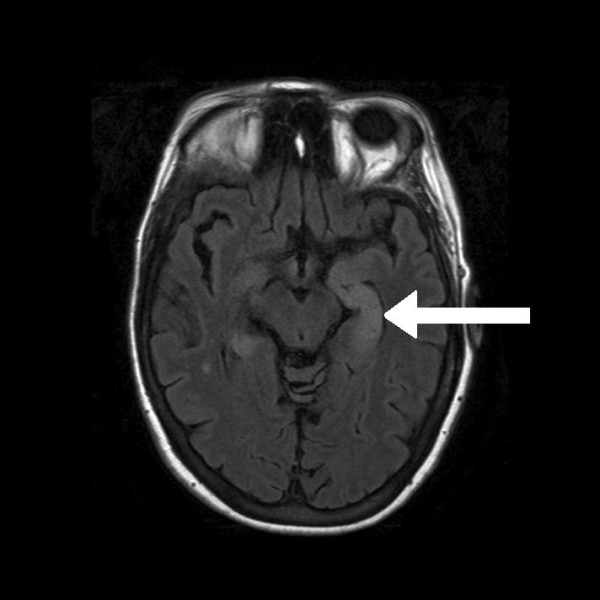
Axial fluid attenuation inversion recovery (FLAIR) MRI of the brain showing a bright signal from the medial temporal lobe on the left side (arrow) consistent with limbic encephalitis.
